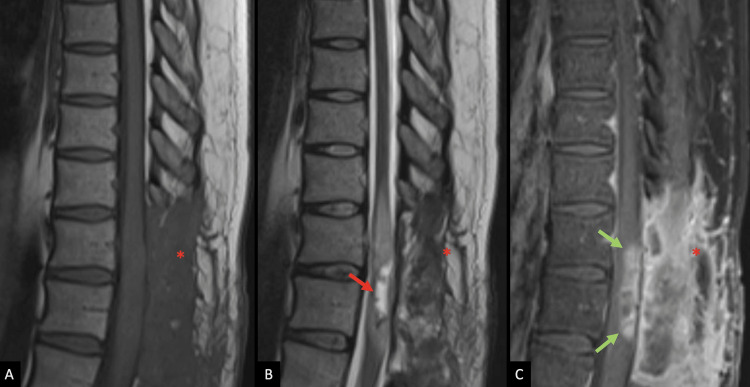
Postoperative MR imaging An MRI of the thoracic spine, approximately two months following the initial preoperative MRI in 2020. Sagittal (A) T1 weighted image (T1WI), (B) T2 weighted image (T2WI), and (C) fat-suppressed, post-contrast T1WI demonstrate changes following posterior decompression at the T10-L1 levels and subtotal resection of the intramedullary tumor. There is T2 hyperintense cystic change at the resection site (red arrow) with surrounding residual, enhancing tumor (green arrows). The extent of vasogenic edema has significantly decreased since the preoperative exam. There are expected post-surgical changes in the dorsal soft tissues (red asterisks).
